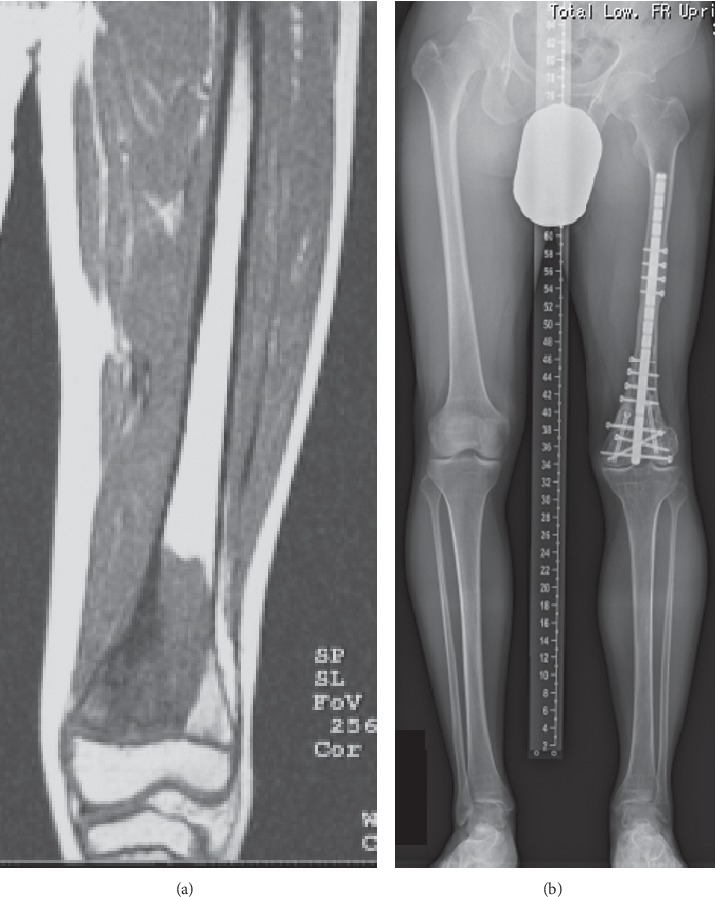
Case #23: 13-year-old male with osteosarcoma of the left distal femur. (a) Preoperative MRI. (b) 21 years after treatment with osteoarticular extracorporeal irradiated autograft. Collapse of the graft was not observed, and he did not undergo reoperation. Growth of the left tibia is preserved. The length of the left and right tibia is similar. The leg length discrepancy is 57 mm.
